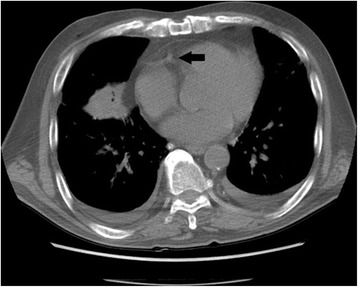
Delayed chest computed tomography scan, performed after 10 min, demonstrating total resolution of the air embolus in the right coronary artery
